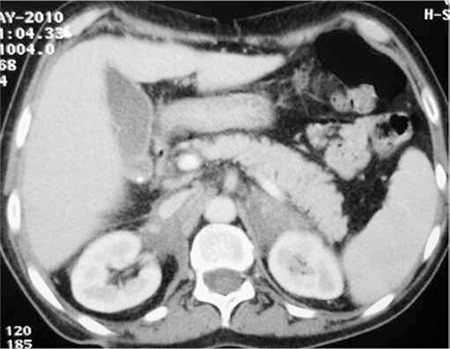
CT scan after 4 cycles of chemotherapy showed partial response.
